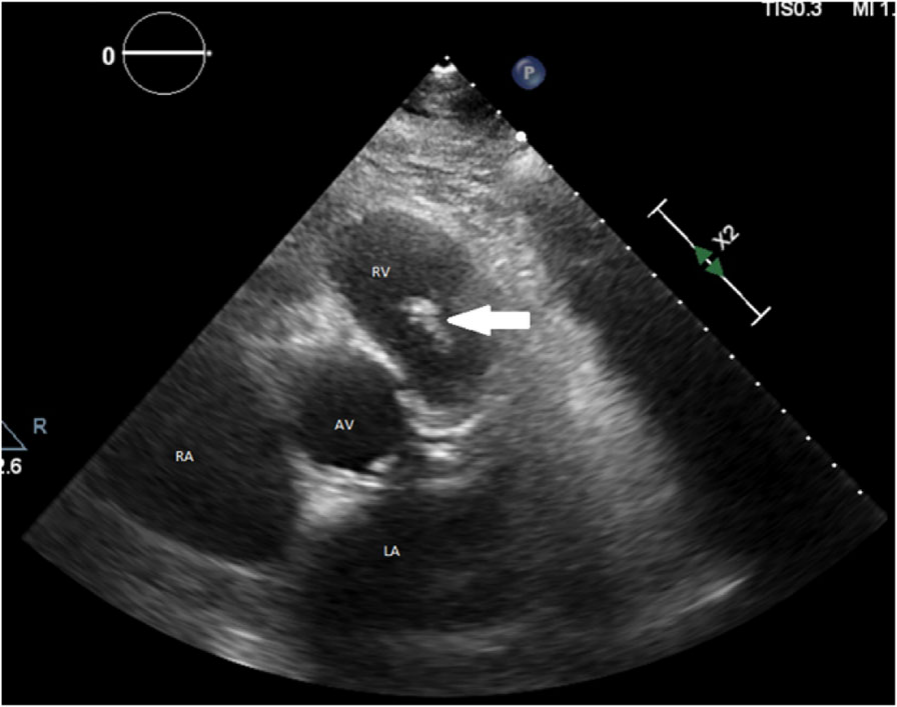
Transthoracic echocardiography parasternal short-axis view revealing a large pulmonary valve mass (*arrow*). *AV* Aortic valve, *LA* Left atrium, *PA* Pulmonary artery, *RA* Right atrium, *RV* Right ventricle
